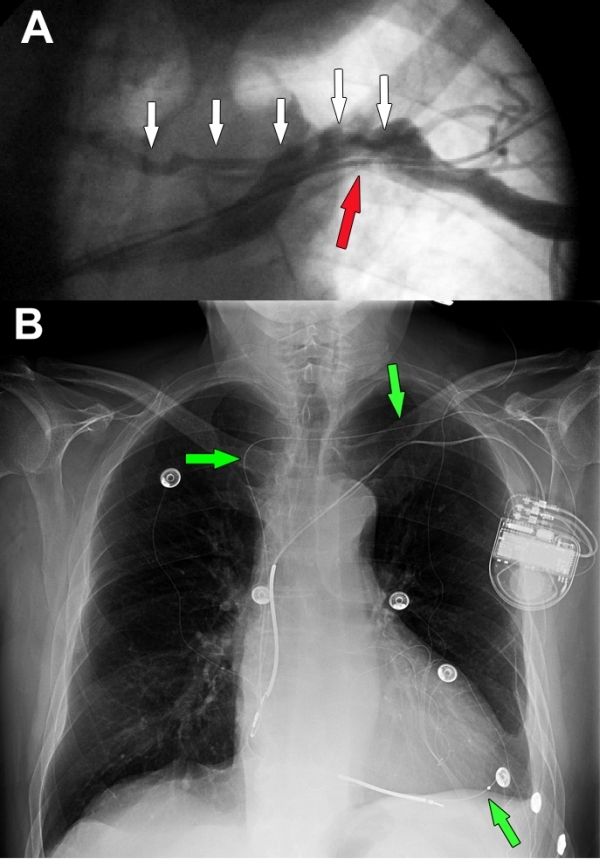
A. Venogram, performed with contrast injection in the left basilic vein, demonstrating a suboccluded left subclavian vein (red arrow) and the presence of a collateral vein (white arrows) draining into the right subclavian vein. B. Chest radiograph (anteroposterior view) obtained the day after the procedure. The coronary sinus pacing lead (green arrows) passes from the left subclavian vein to its final position via a collateral vein and the right subclavian vein. The CS lead is placed in a posterior location.
